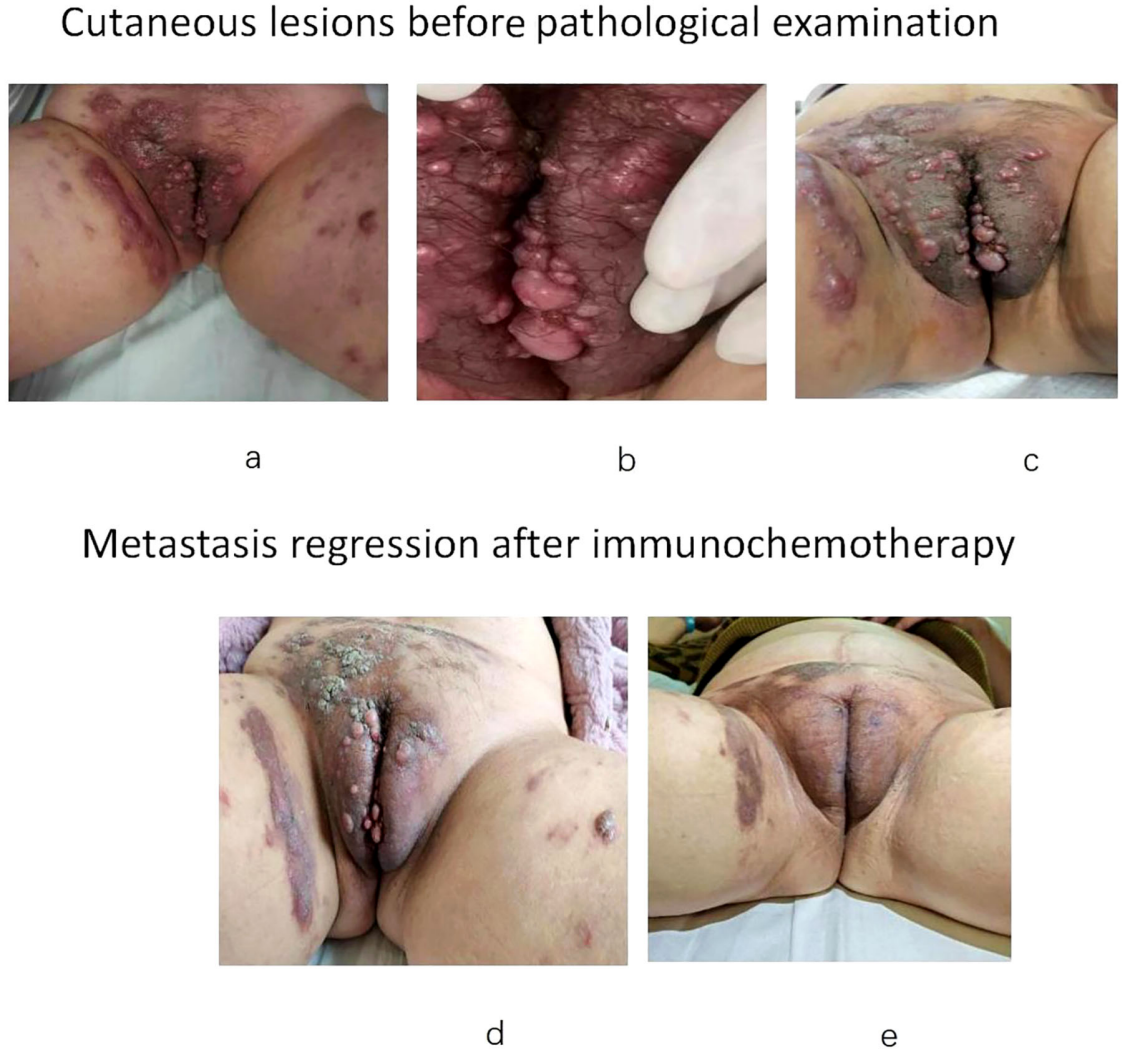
(A) Shows the red herpes-like solid papules and nodules of rice grain to soybean size on the pubic mound, vulva, labia majora and minora, the upper third of the left thigh, and the root of the right thigh. (B, C) Show the solid papules and nodules that grew to the size of soybeans or peanuts. (D) Shows the partial regression of skin metastases after the third cycle of changed treatment. (E) Shows the complete regression of skin metastasis after the fourth cycle of changed treatment, and CT confirmed the regression of inguinal lymph nodes.
